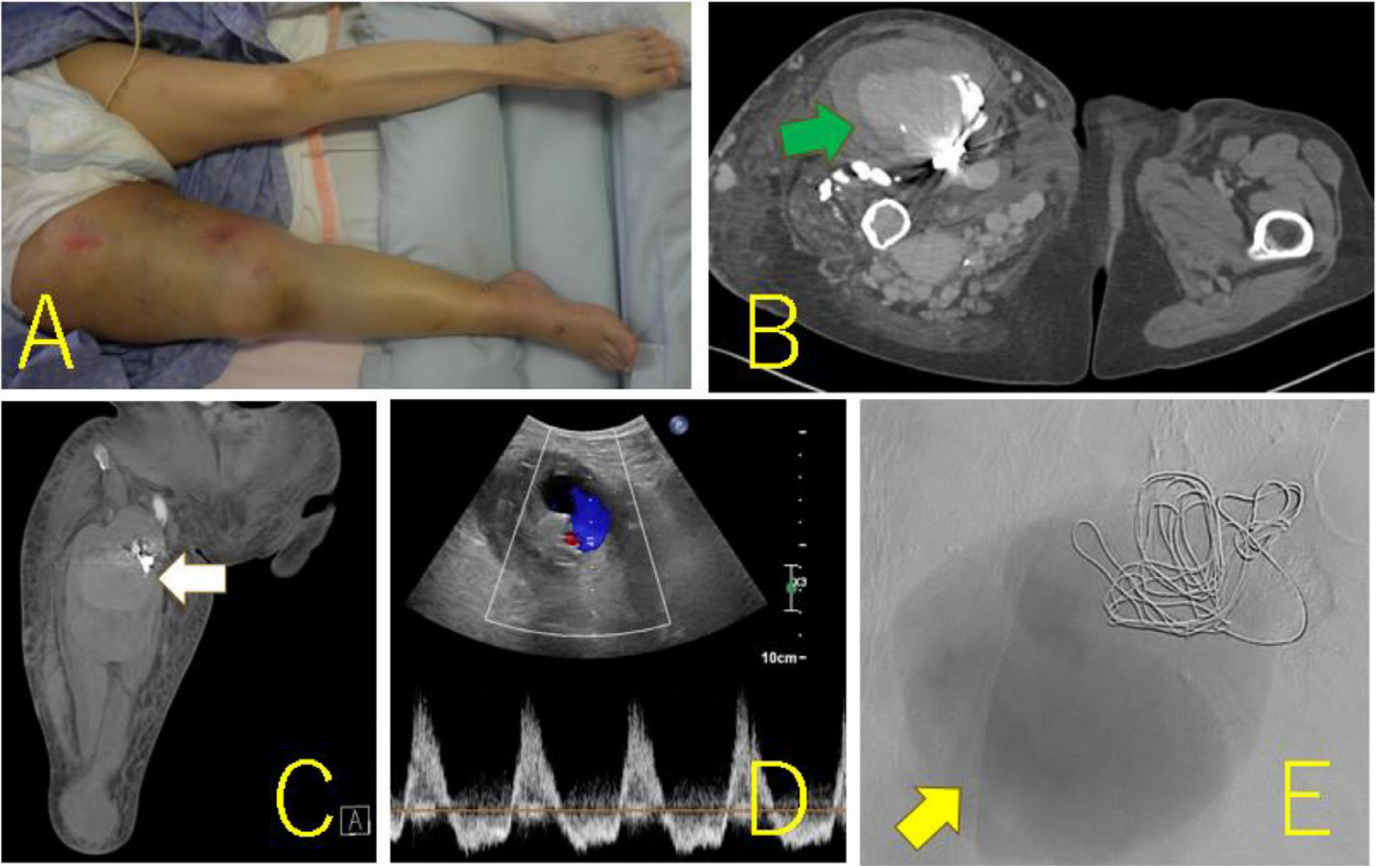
The photograph and imaging findings on admission to our hospital. (A) The right thigh was swollen, was twice as large as the left leg, and had red skin lesions. (B, C) Axial (B) and coronal (C) contrast-enhanced computed tomography revealing a giant aneurysm (green and white arrows) (97 × 71 × 154 mm) next to the coils. (D) Ultrasonography of the limb arteries shows a to-and-fro waveform between the giant aneurysm and the coiled B2 arterial aneurysm, suggesting that the giant aneurysm was a pseudoaneurysm. (E) Digital subtraction angiography image showing the pooling of the contrast agent in the giant pseudoaneurysm (yellow arrow).
